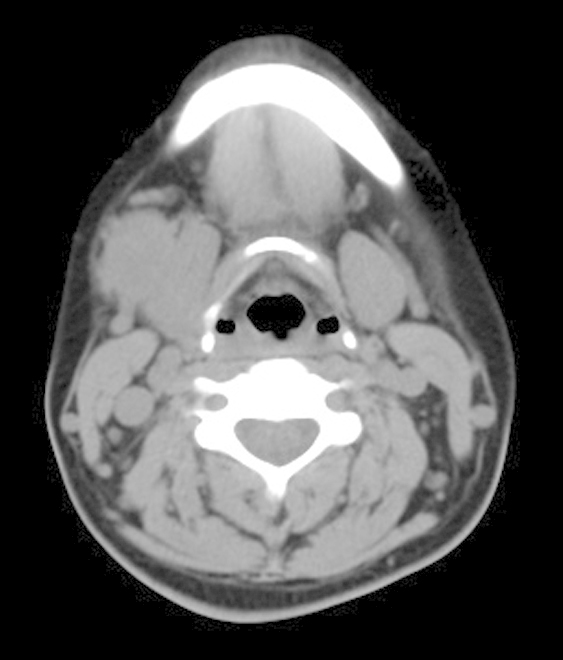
Computed tomography scan of a soft-tissue mass located adjacent to the right submandibular gland.
